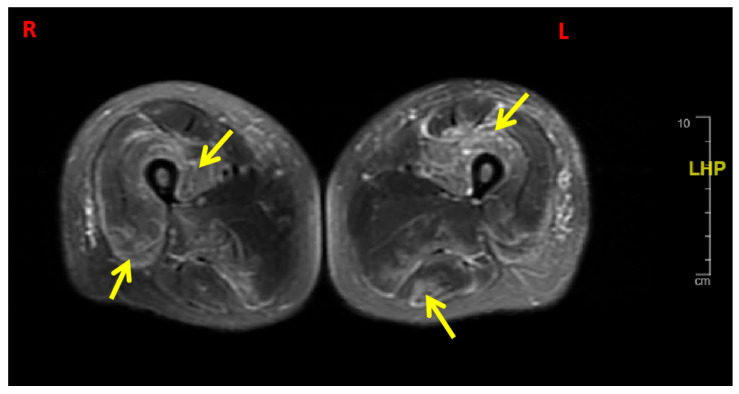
Magnetic resonance imaging of the right (R) and left (L) thighs, T2-weighted axial images. Images reveal diffuse hyperintensity and edema involving the quadriceps and hamstrings, consistent with active myositis.
